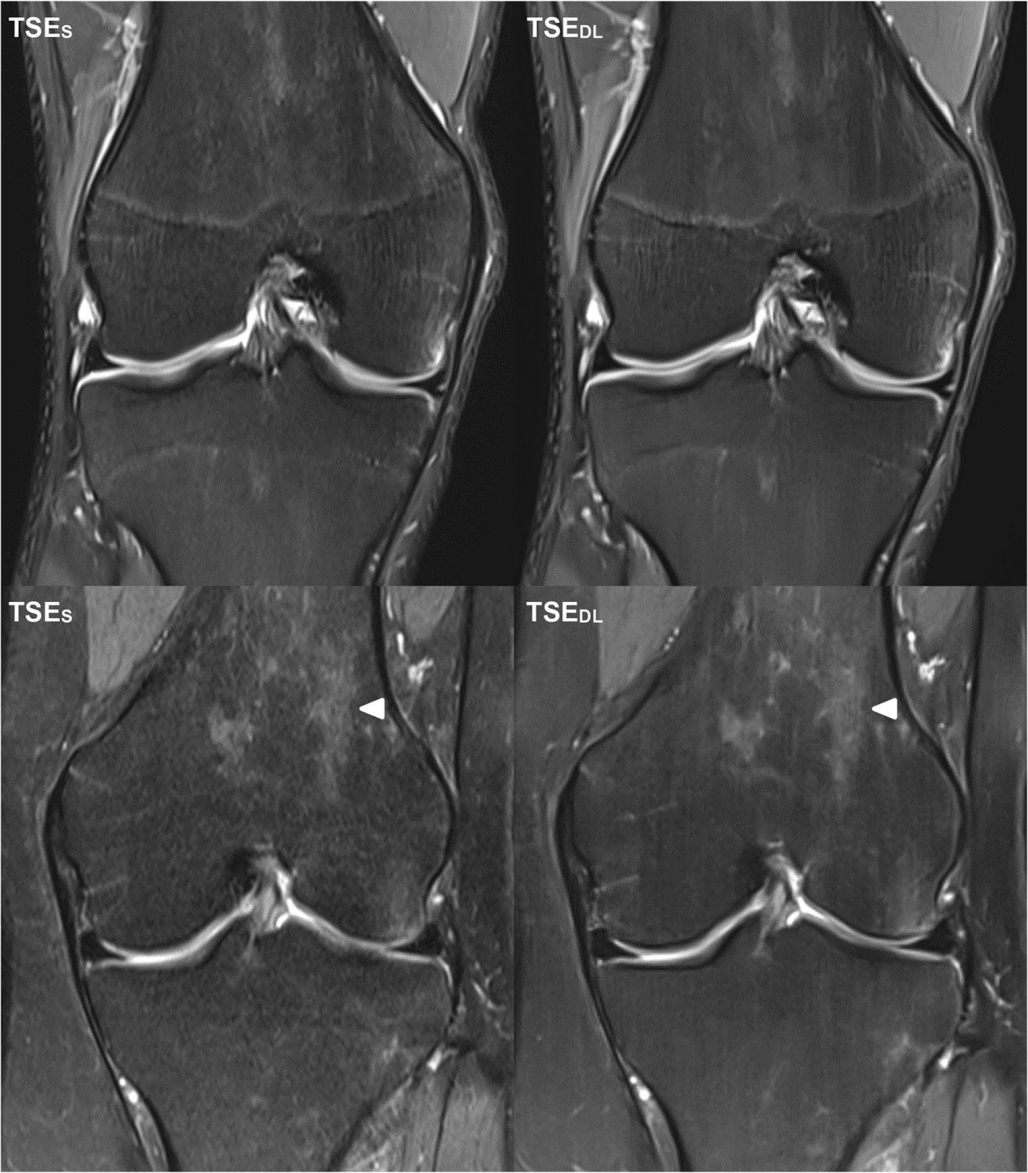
Image examples of a standard and deep-learning-reconstructed PD TSE imaging of the knee at 3 T and 1.5 T. The upper-row images are examples of knee MRI at 3 T in coronal orientation of an 18-year-old professional athlete with pain in the area of the patella of both knees. After a break from training, the complaints had improved. The lower-row images are examples of knee MRI at 1.5 T in coronal orientation (lower row, PD TSE_S_ left and PD TSE_DL_ right) of a 30-year-old patient after knee distortion. Comparing PD TSE_S_ (left) and PD TSE_DL_ (right), in PD TSE_DL_, the difference in the extents of noise at 3 T (upper row) is less present than in images acquired at 1.5 T (lower row). Unfortunately, TSE_DL_ images at 1.5 (lower row, right) show characteristic banding artifacts (white arrowheads), known as streaking, which are not present in TSE_S_
